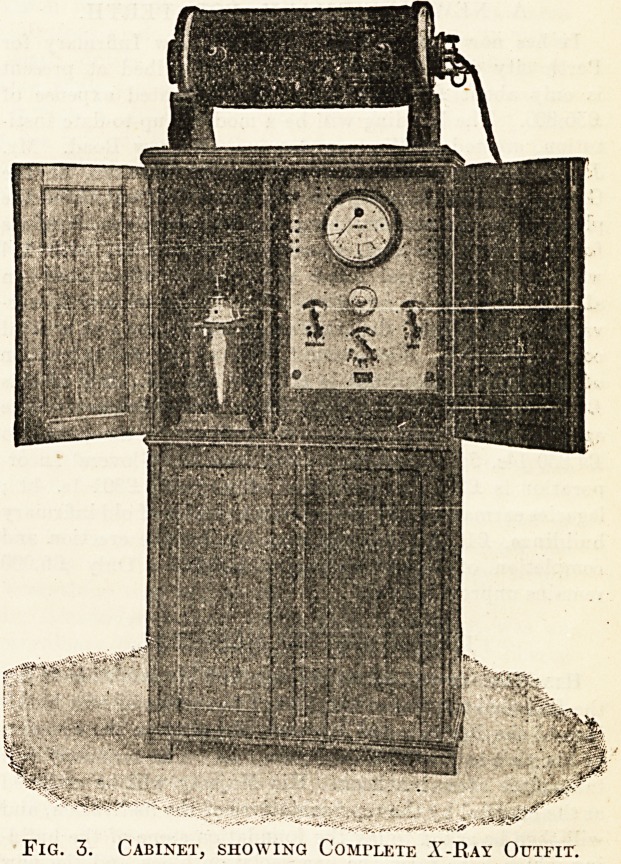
# Modern X-Ray Apparatus
